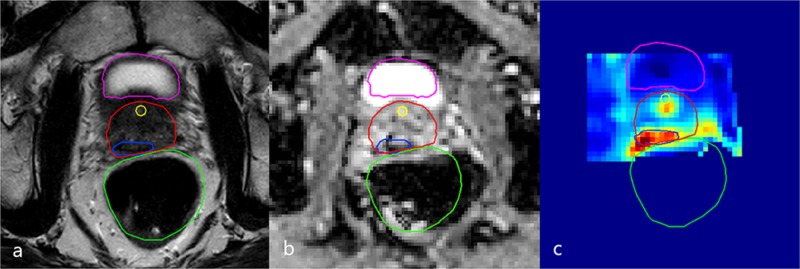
Diagnostic 3T mp-MRI revealing first recurrence lesion Transversal plane of the diagnostic 3T multiparametric-magnetic resonance imaging (mp-MRI) revealing a suspect lesion in the right dorsal peripheral zone next to the prostate midline and in the right seminal vesicle. Delineations of the bladder (purple), urethra (yellow), rectum (green), prostate (red), and gross tumor volume (GTV, blue) are shown. In this case, the clinical target volume (CTV) was considered equal to the GTV because there was mainly seminal vesicle invasion. (a) T2-weighted image, (b) ADC image, (c) K-trans image
